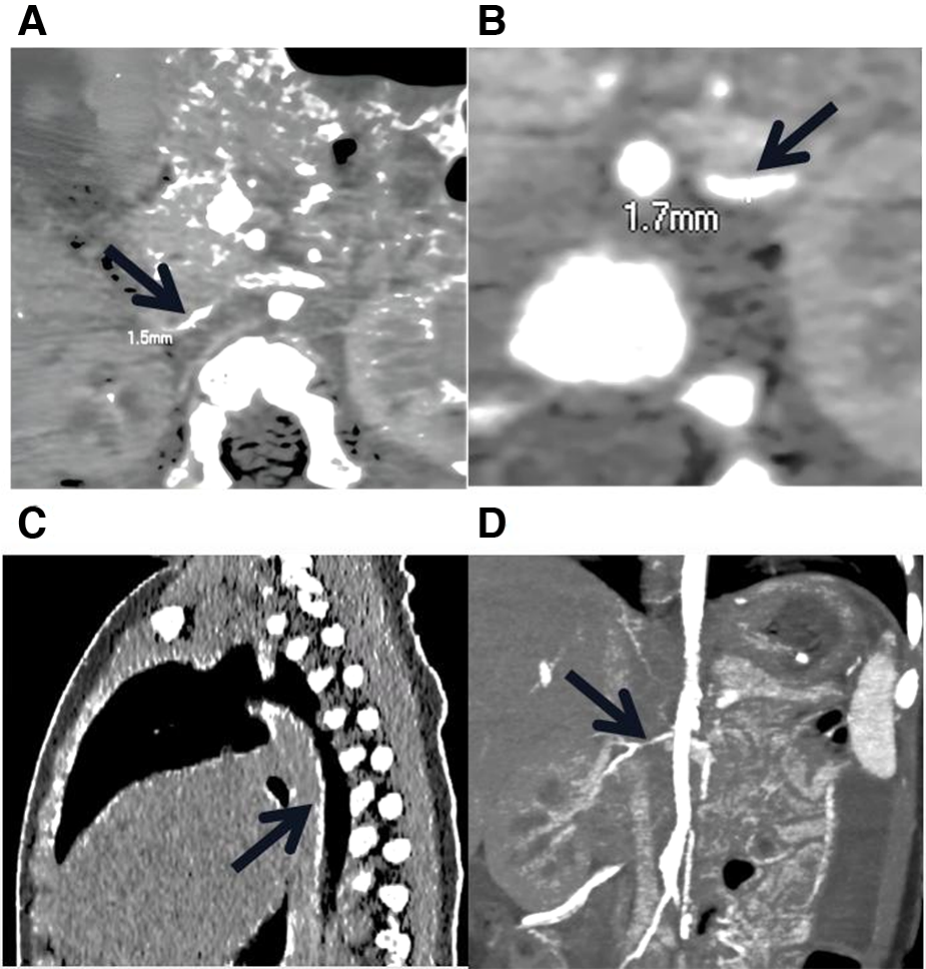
Low-dose, enhanced CT of the child with GACI caused by a homozygous variation in *ENPP1*. (A) and (B) stenosis of bilateral iliac artery; the thinnest parts of the left and right iliac arteries are 1.7 mm and 1.3 mm wide, respectively. (C) The black arrow indicates the presence of calcification in the descending aorta, thoracic aorta, and abdominal aorta. (D) The black arrow indicates the stenotic right renal artery.
